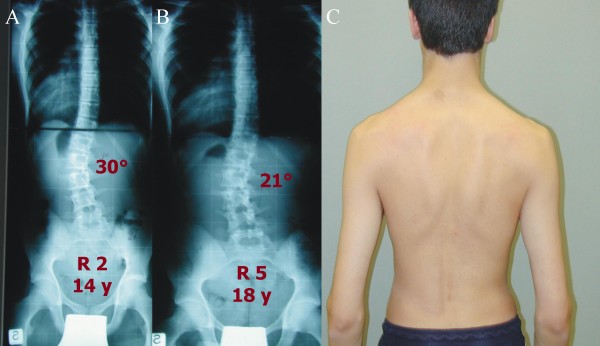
Results of a male patient. A. Starting x rays. B. Final x-rays. C. Aesthetic final result. C decreased from 30° at 14 years of age, Risser 2 to 21° at 18 years, Risser 5.
